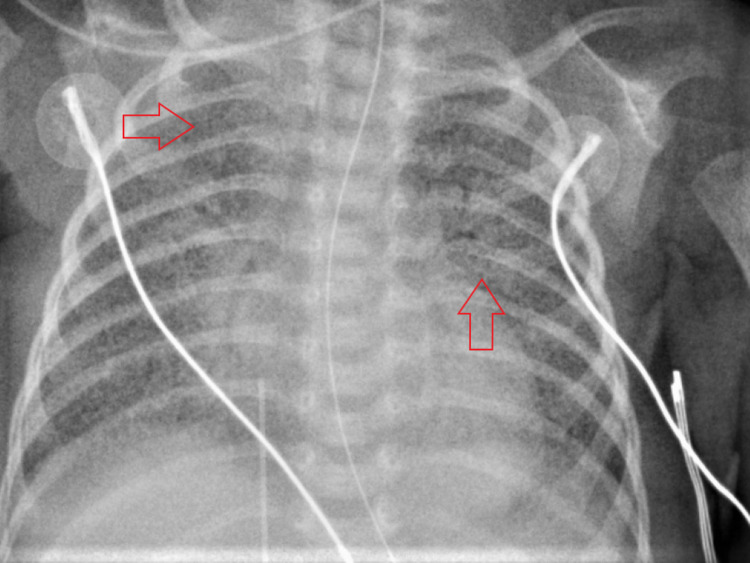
CXR from the last day of life show coarse, bilateral, parenchymal infiltrates (arrows) CXR - chest x-ray
